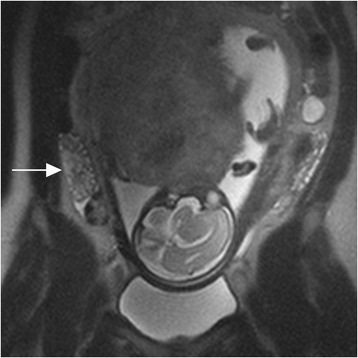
Ovarian torsion without stimulation. Coronal T2-weighted image in a 30-year-old woman with right lower quadrant pain at 28 weeks gestational age demonstrates an enlarged right ovary (arrow) with increased T2 stromal signal intensity and a normal appearing left ovary. The right ovary was torsed 720 degrees and was surgically removed
